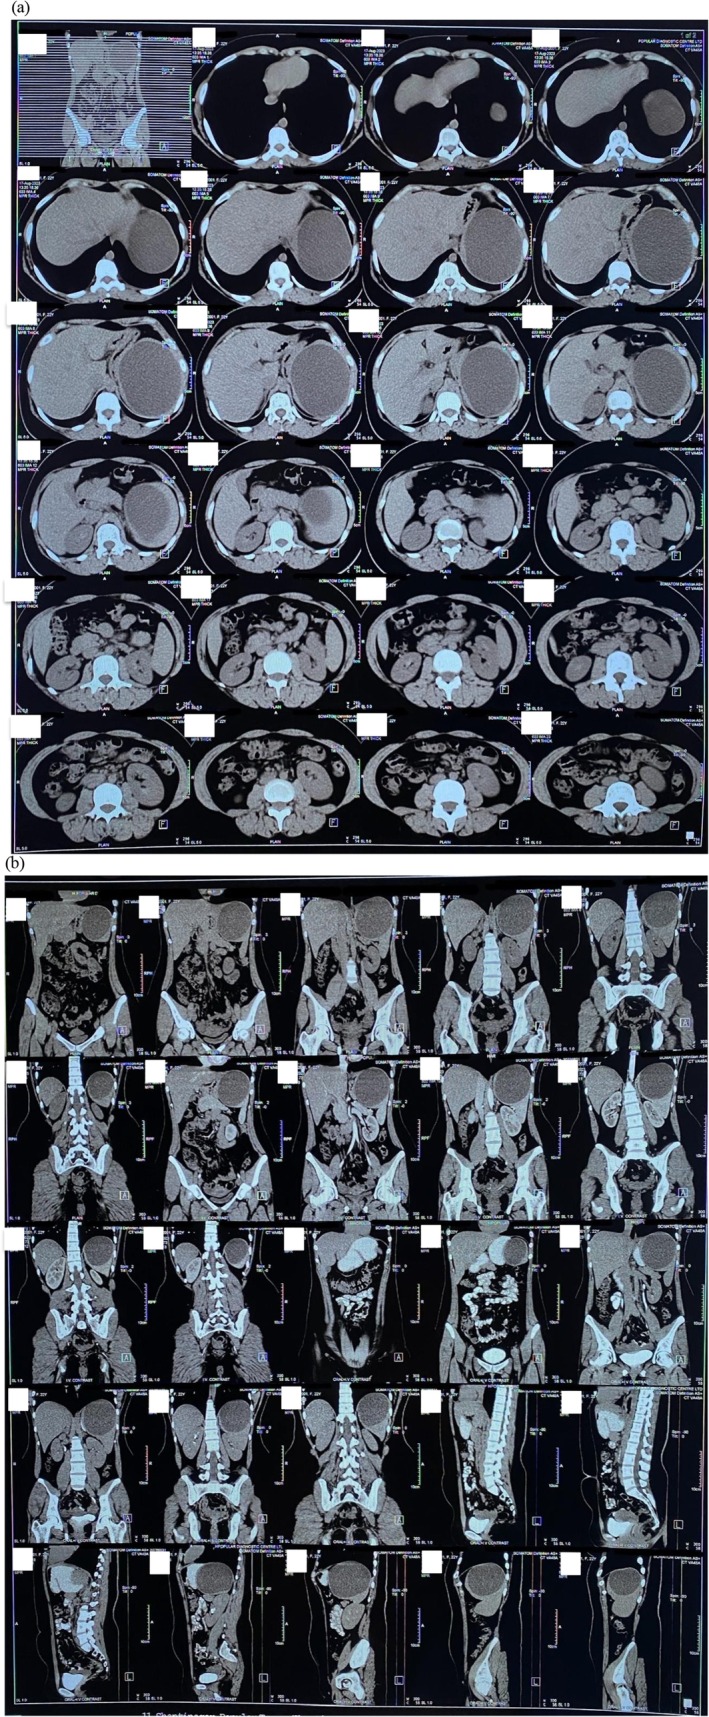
Plain and contrast (a) transverse section and (b) coronal section CT scan of abdomen showing a large cyst in the upper pole of spleen measuring 9.6 cm in AP, 12.2 cm in TR & 11.8 cm in CC. Note that there is no post‐contrast enhancement.
